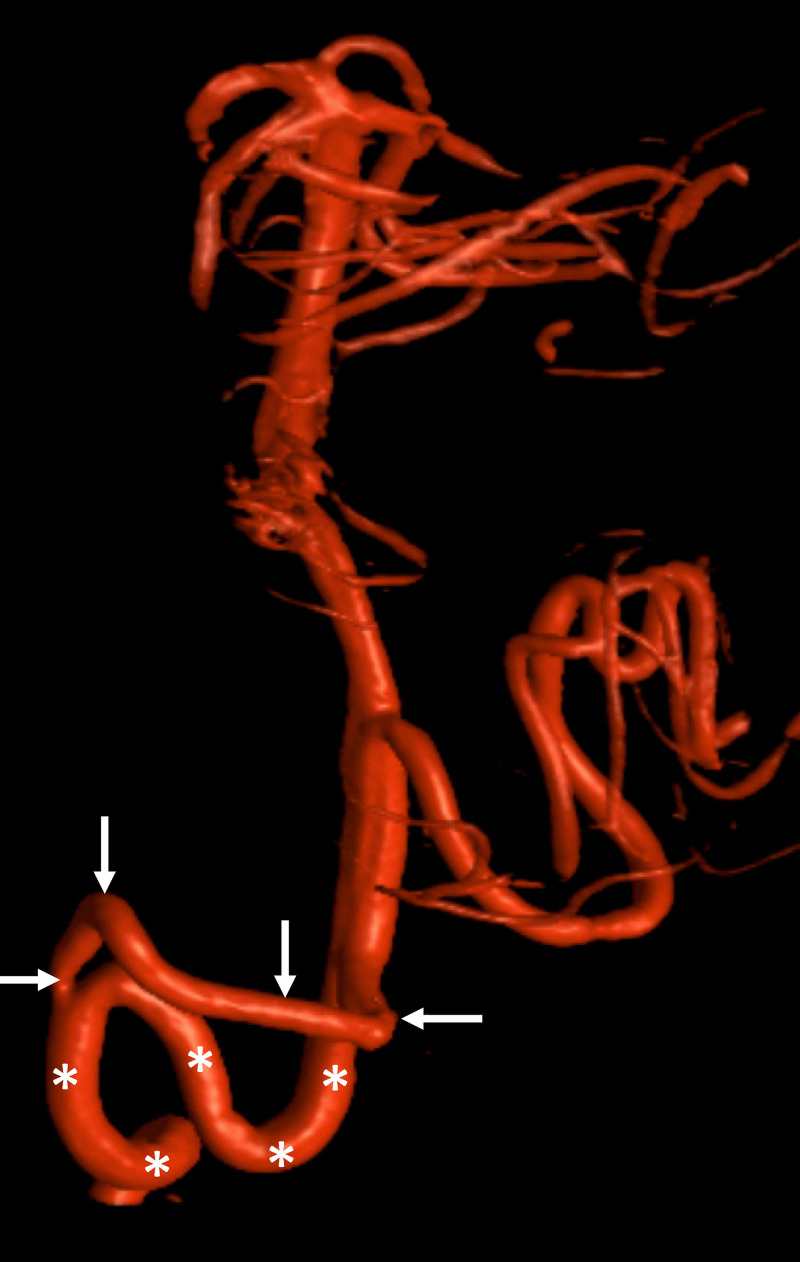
CTA noting a duplicated vertebral artery (arrows) with normal vertebral artery (asterisks) CTA: CT Angiogram
